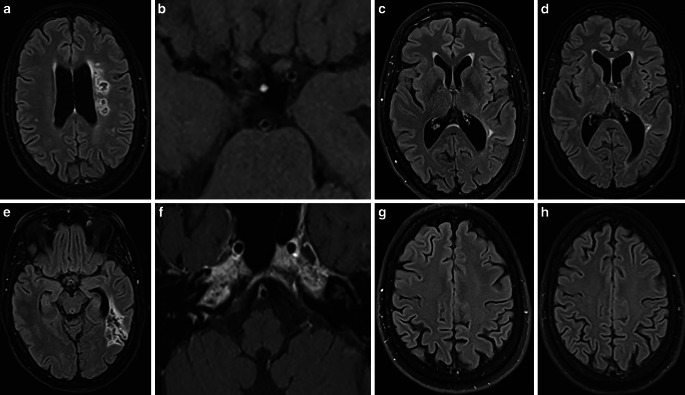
Follow-up MRI scans after stent angioplasty for intracranial stenoses secondary to a LNB-associated cerebral vasculitis. FLAIR images (axial views; a, e) and fat-saturated contrast-enhanced T1-weighted images (axial views; b, f) after 3 months demonstrating unchanged chronic infarct areas in the left temporal lobe and in the left semioval center, and regressed mural thickening and contrast enhancement in the intracranial arteries. FLAIR images after 6 months (axial views; c, g) and 12 months (axial views; d, h) showed no new cerebral lesions
